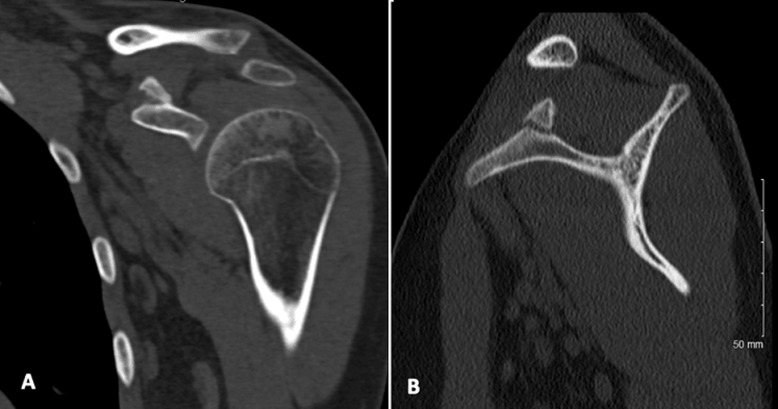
CT-scan views 3 months post operatively, A) coronal view; B) sagittal view
